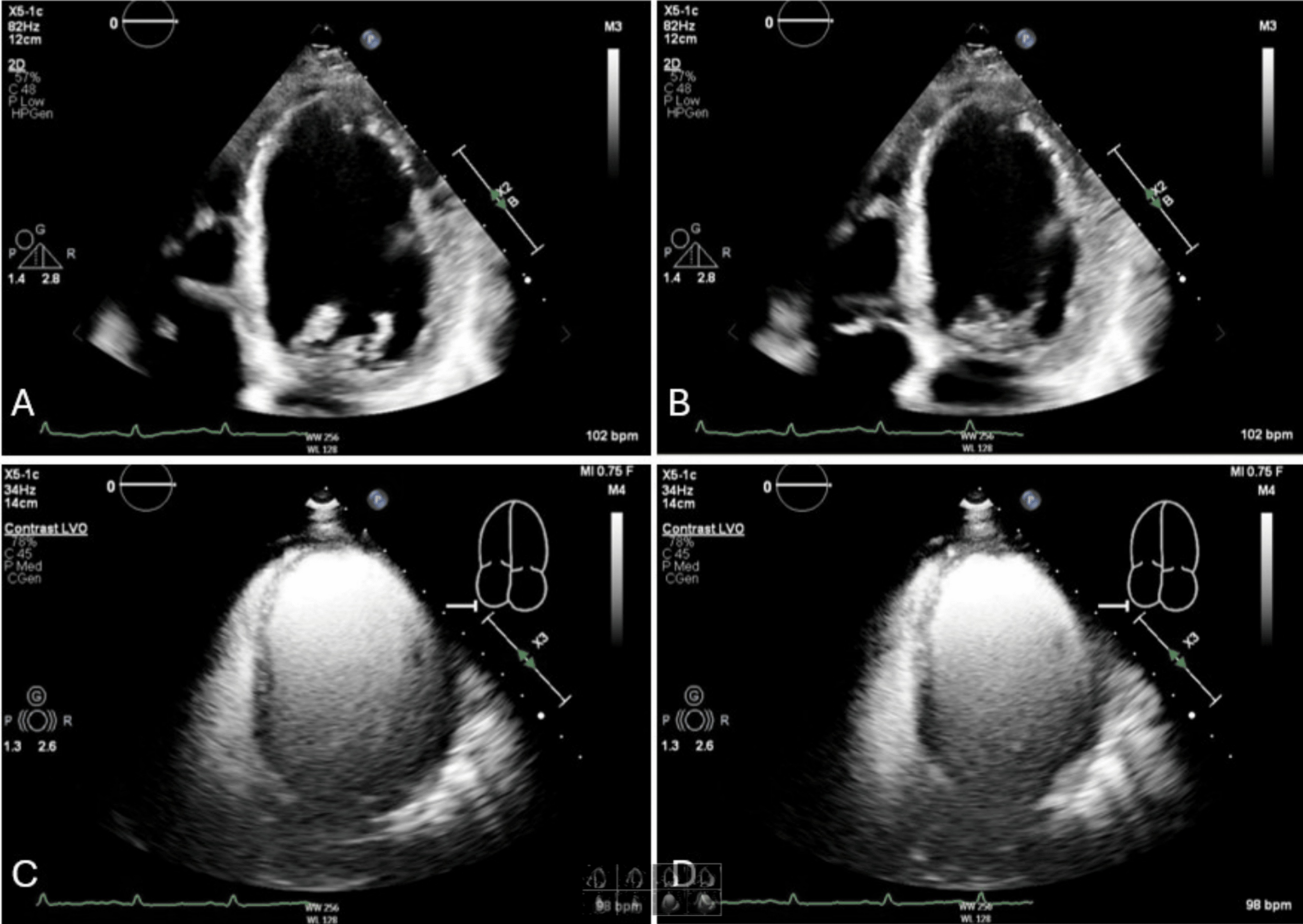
Echocardiogram images of the apical four-chamber view during (A) diastole and (B) systole, as well as left ventricular opacification views during (C) diastole and (D) systole, demonstrate a severely reduced left ventricular ejection fraction (10–15%) prior to doxycycline treatment.
